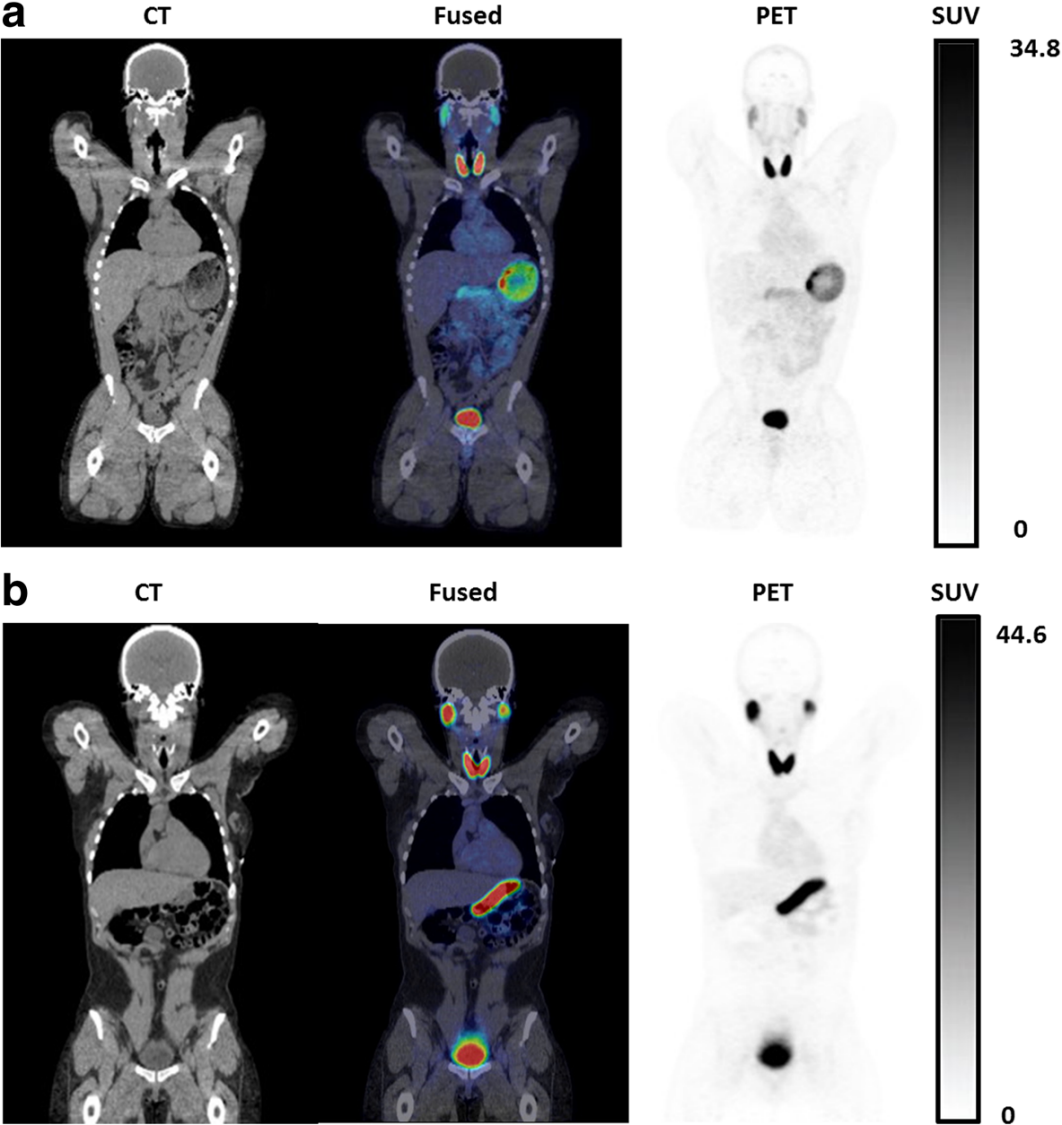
Coronal PET/CT images of ^18^F-TFB in healthy male (a) and female (b) participants at 2 h post-injection. Physiologic distribution of ^18^F-TFB is seen in the thyroid, salivary glands, stomach, and intestines. Prominent excretion of radioactivity is seen in the urinary bladder
